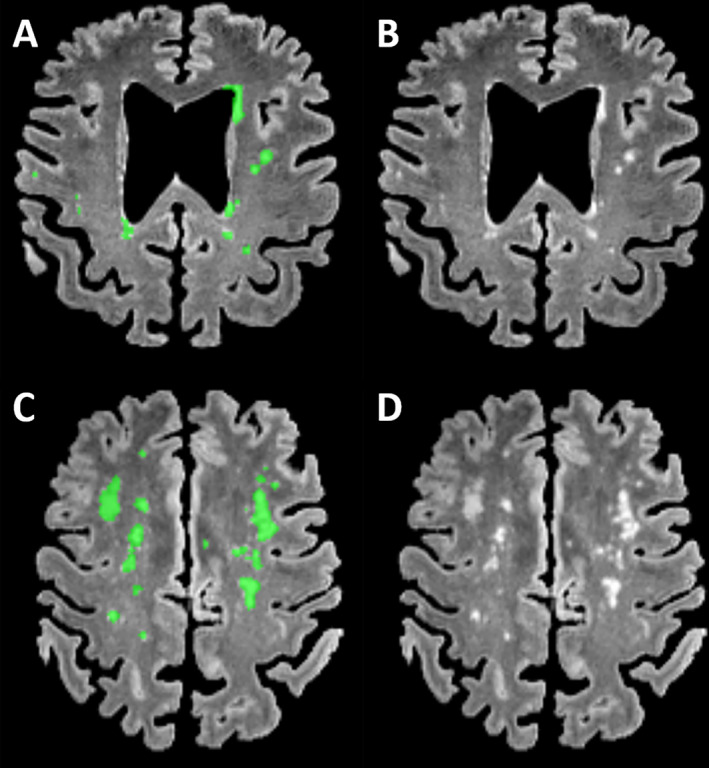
Axial FLAIR reformats in patients (A/B, 67 year‐old male; C/D, 75 year‐old female) with corresponding superimposed segmentations of white matter lesions (B, 4.8 ml total lesion load; D, 17.8 ml total lesion load).
